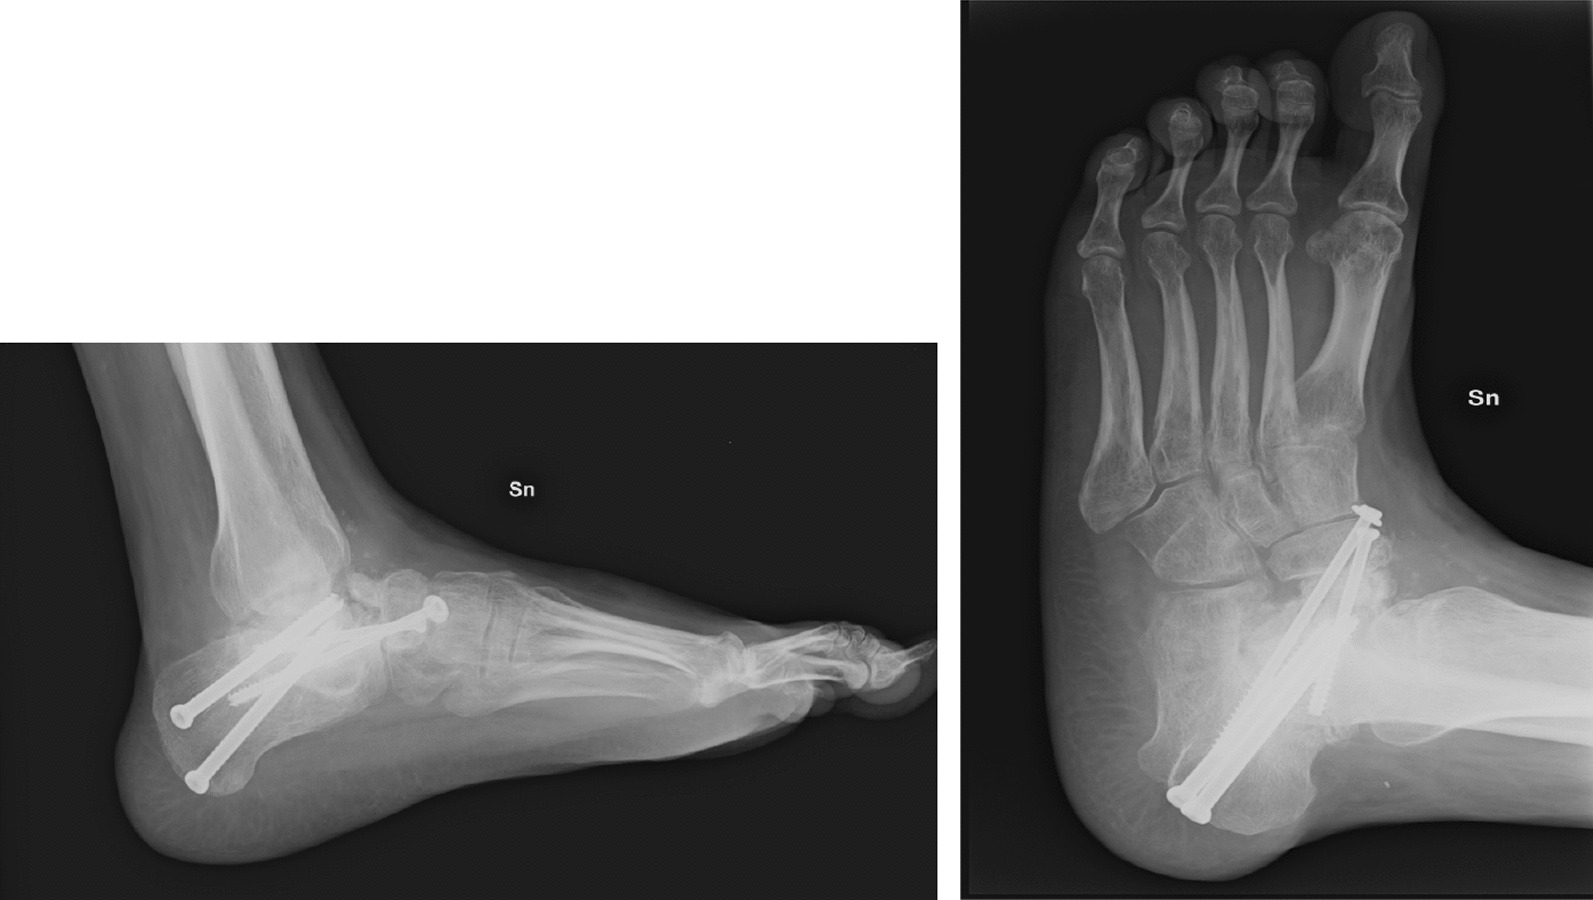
4 months post-operative x-ray
